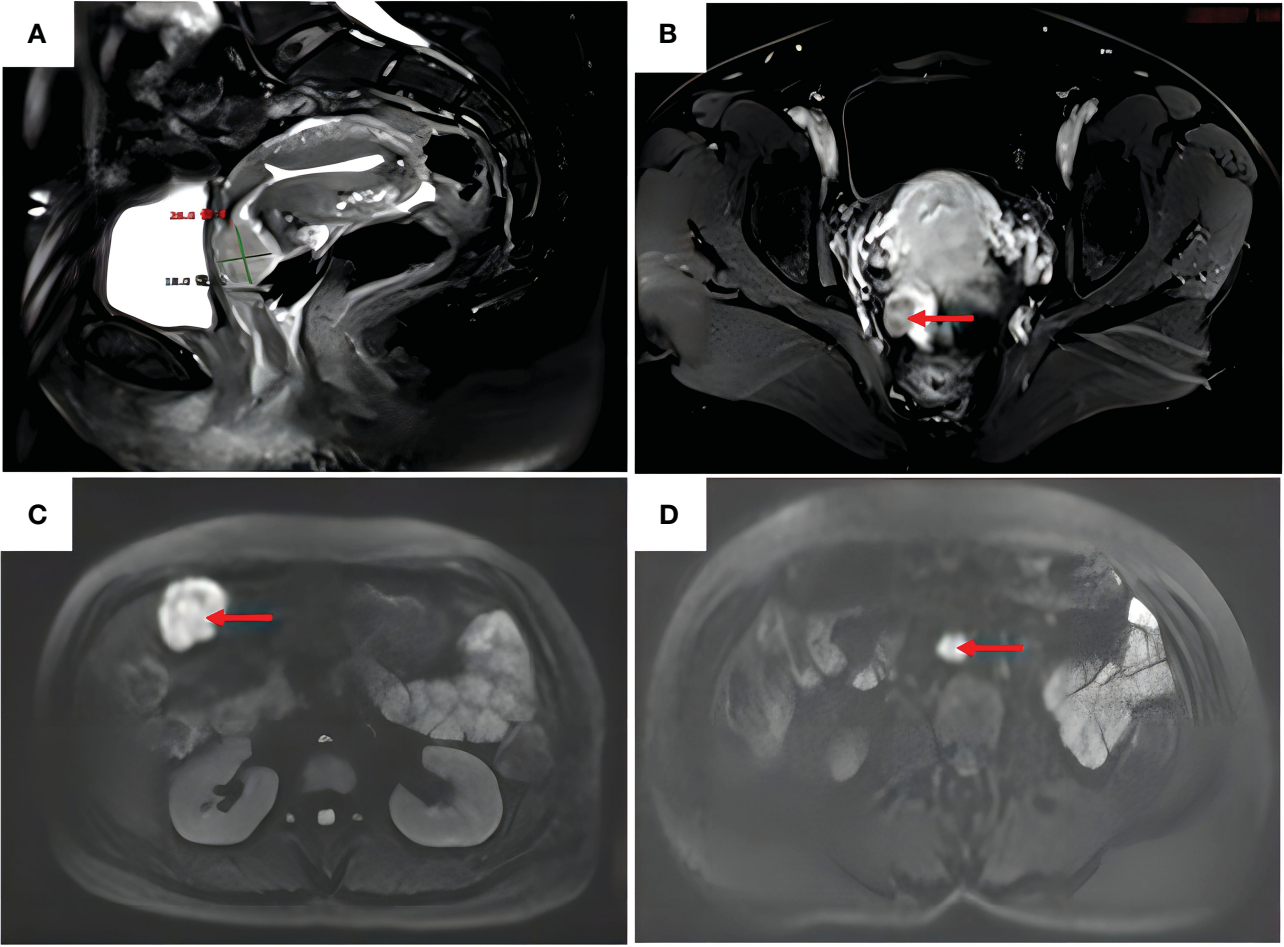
Full abdomen + pelvis magnetic resonance imaging. (A) Anterior cervical lip with a slightly enhanced signal on T2–weighted images, approximately 26 mm in diameter. (B) Enhancement of the right pelvic nodal lesion (red arrow) on T1–weighted images. (C) Enhanced signal of the mass in the right upper abdomen (red arrow) on diffusion–weighted images. (D) Enlarged retroperitoneal abdominal para–aortic lymph nodes (red arrow) on diffusion–weighted images.
